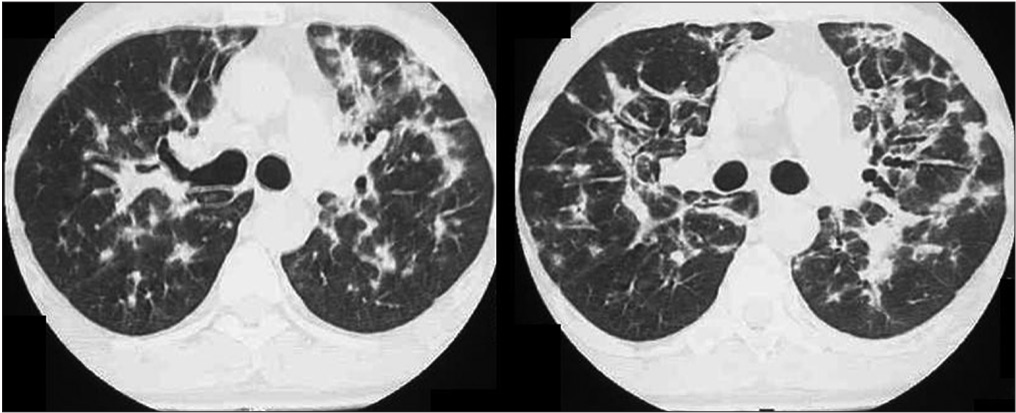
Bronchiolitis obliterans with or without organizing pneumonia in the absence of infection can be a feature of acquired immunodeficiency syndrome (AIDS). This is an infrequent imaging diagnosis, although focal air trapping on expiratory computed tomography, consistent with bronchiolitis obliterans, has been demonstrated in two-thirds of human immunodeficiency virus-positive patients without AIDS, the severity increasing with the duration of infection
